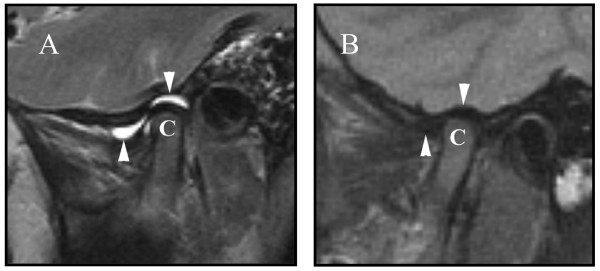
Image A is a T2-weighted proton density parasagittal MR image through the right TMJ showing effusions (arrows) just anterior to the condyle (C) in the superior and inferior synovial spaces. Follow up T2 MR image 4 weeks later (image B) is normal.
